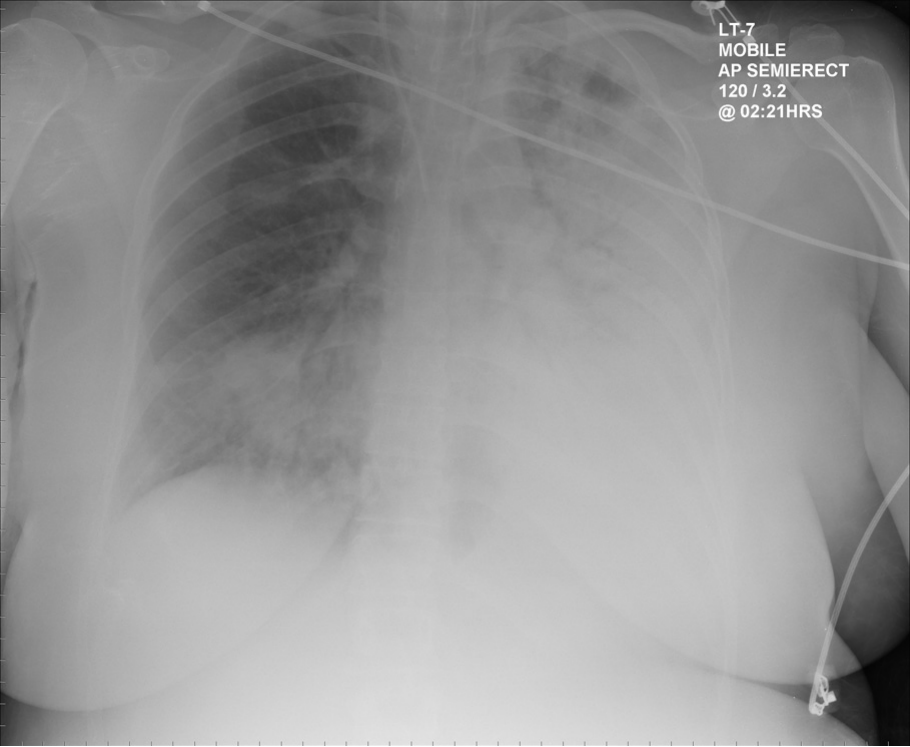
Chest radiograph of our first patient demonstrating an infective infiltrate.
